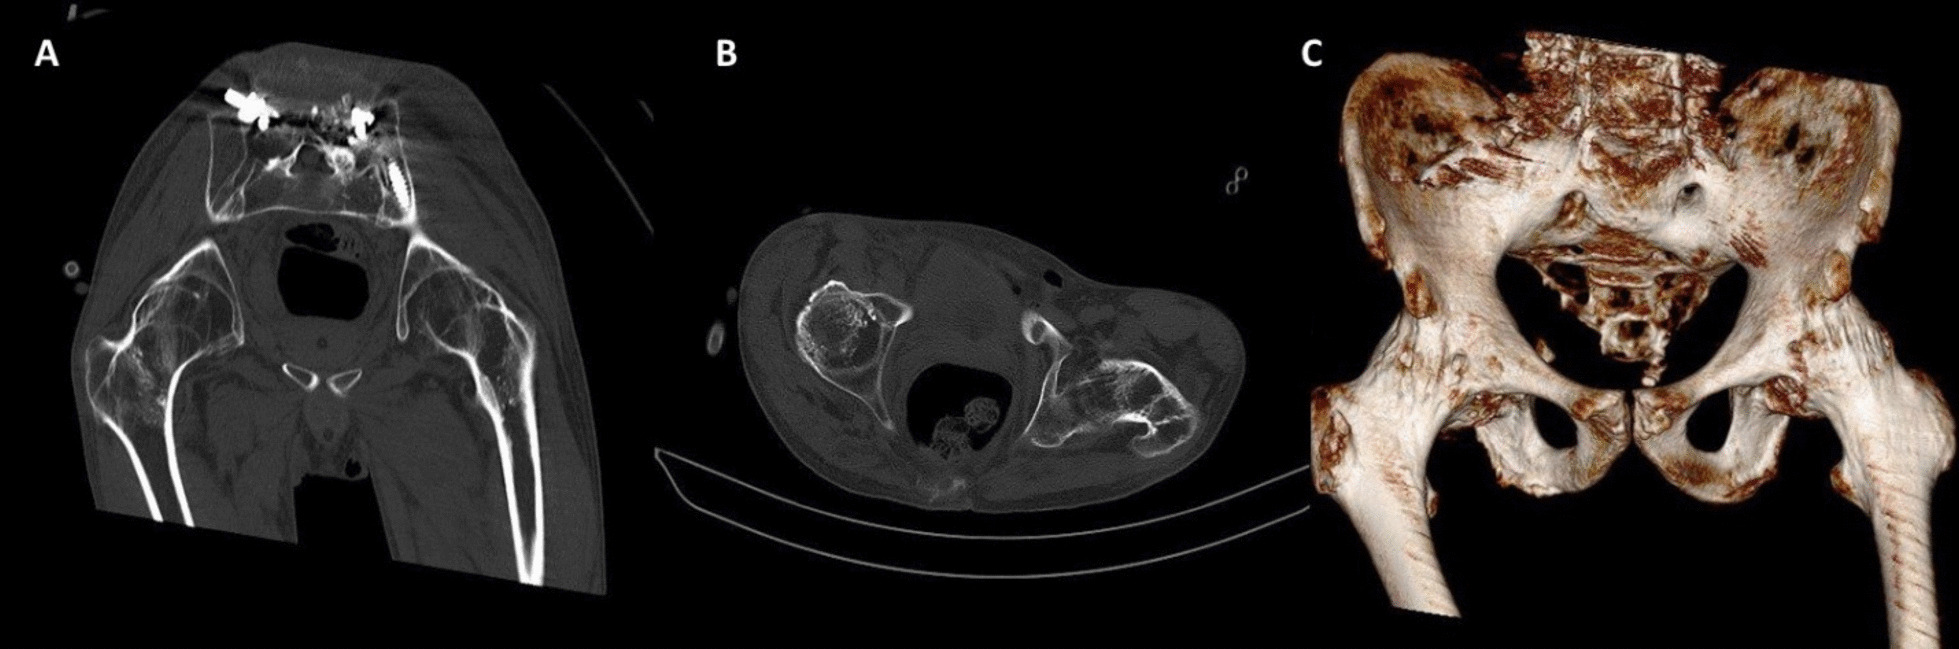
Computed tomography evaluation of the hip in A coronal and B Axial planes, and C with further 3 dimensional reconstruction
